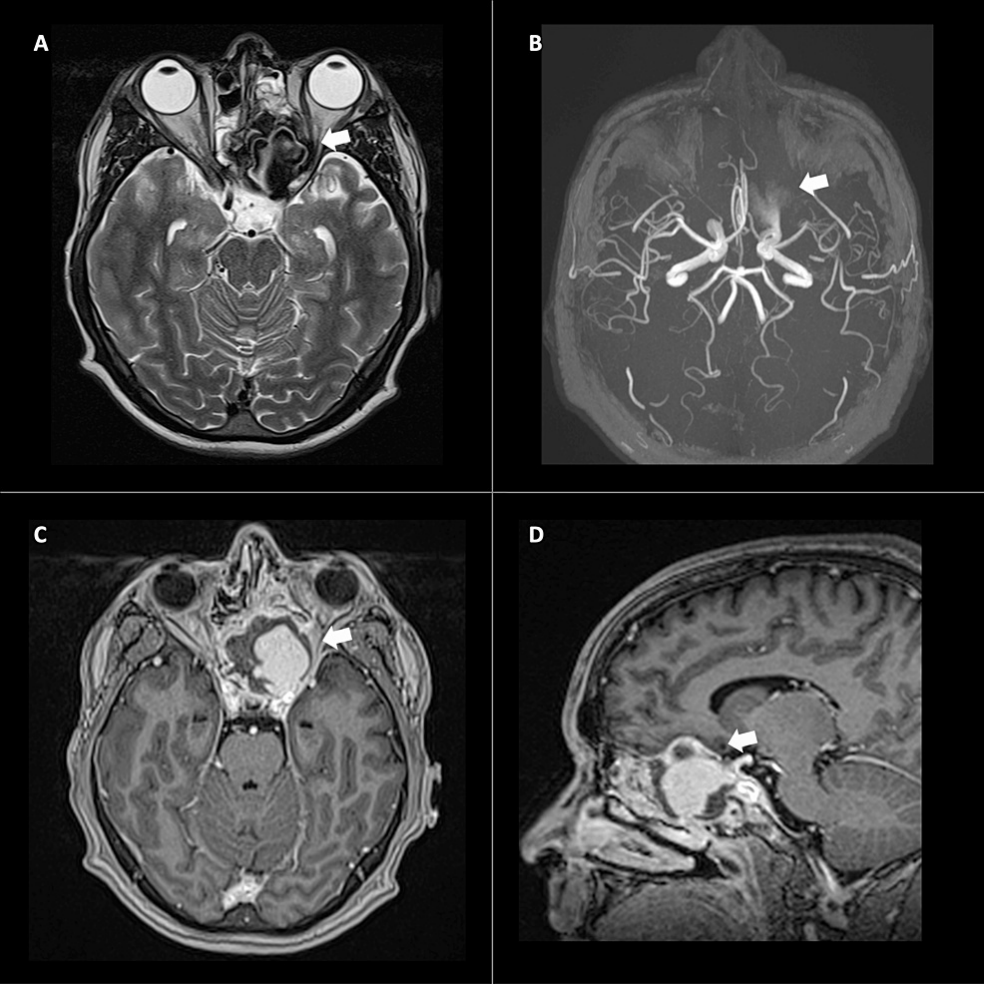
Contrasted MRI of the brain. A. Axial T2-weighted. A well-defined heterogeneous lesion is present in the left sphenoid sinus, showing partial signal void connecting to the ophthalmic portion of the left ICA. B. TOF MPR. The lesion is partially defined by partial signal void, likely caused by slow flow. C. T1-weighted post gadolinium axial and D. sagittal. The lesion shows avid enhancement with contrast, indicating continuity with the left ICA. A diagnosis of pseudoaneurysm of the ophthalmic segment of the left ICA was made. ICA: Internal carotid artery
